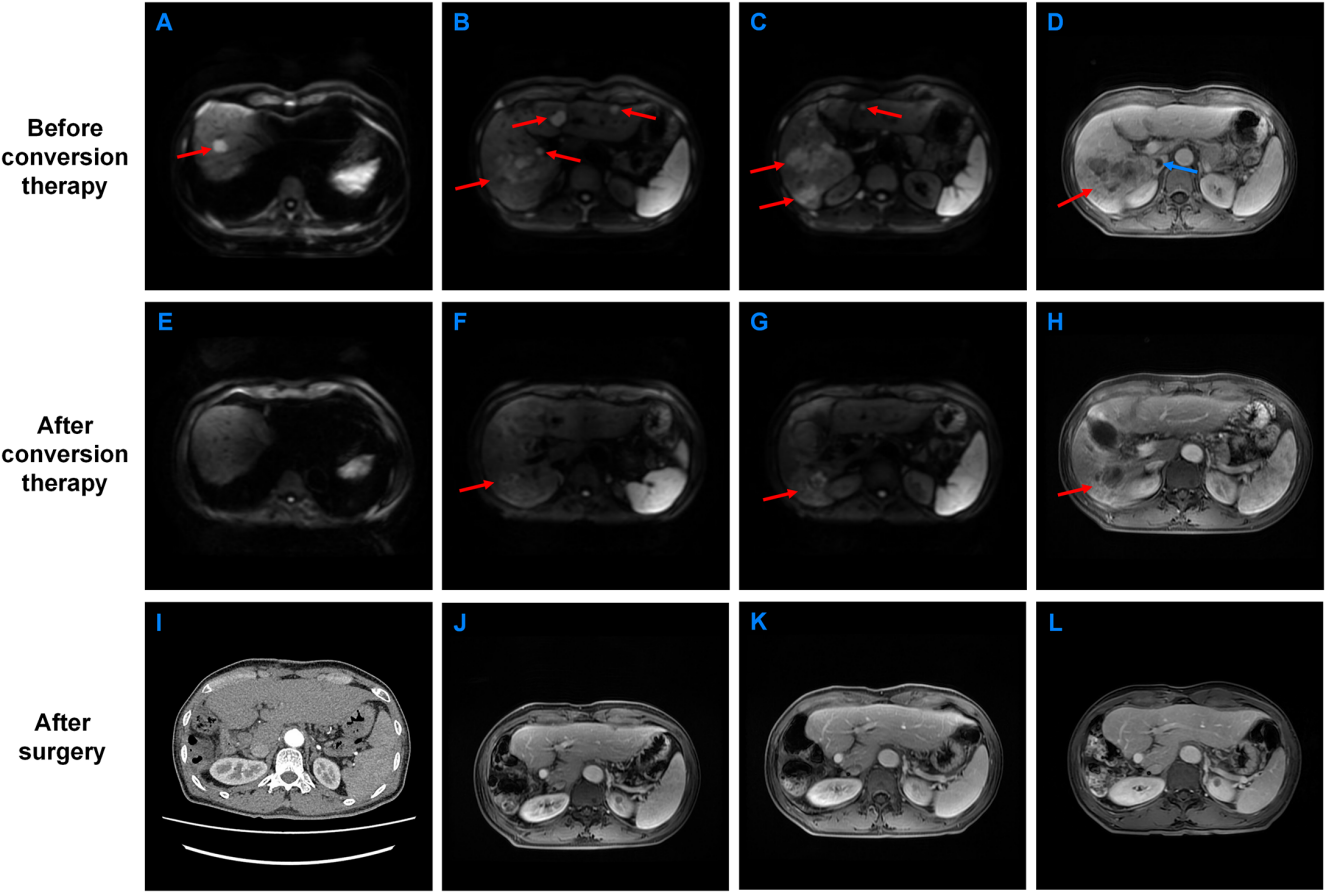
Abdominal magnetic resonance imaging (MRI) and computed tomography (CT) of the patient before and after treatment. (A–C) MRI (DWI) of the patient before conversion therapy. (D) MRI (venous phase) of the patient before conversion therapy. (E–G) MRI (DWI) of the patient after conversion therapy. (H) MRI (venous phase) of the patient after conversion therapy. (I) The patient’s CT at 1 month after surgery. (J) The patient’s MRI at 1 year after surgery. (K) The patient’s MRI at 2 years after surgery. (L) The patient’s MRI at the most recent follow-up. Red arrows represent tumor lesions and blue arrows represent venous tumor embolus.
